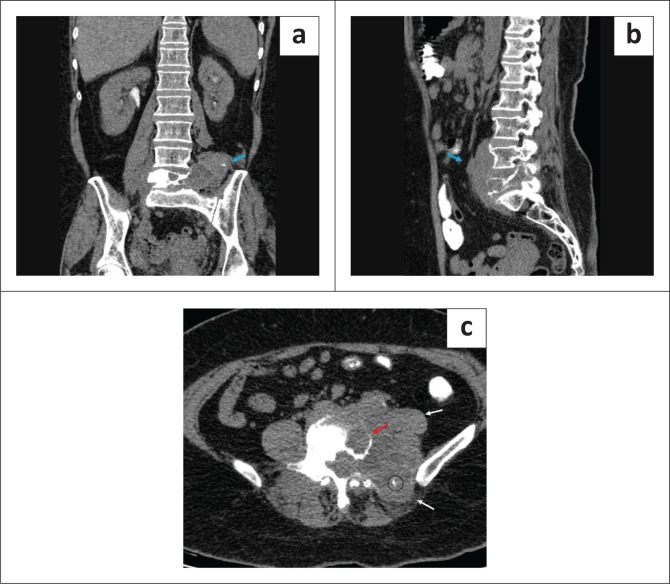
Non-contrast CT scan. An ill-defined soft tissue density lesion is seen along the anterior aspect of the inferior endplate of the L3 vertebra to the anterior aspect of the S1 vertebral body and extending laterally from the L5 vertebral body to the right and left paravertebral regions, predominantly on the left side (blue arrow) (a–b). The L5 vertebral body appears sclerotic and shows an expansile destructive lytic lesion involving the vertebral body with complete destruction of the left lateral vertebral body, left transverse process, left pedicle, left lamina of vertebral arch and left superior articular process (red arrow). Posteriorly, the lesion is seen extending between the left iliac crest and left transverse process of the L4 vertebra and is seen compressing the left posterior paraspinal musculature. Anteriorly, the lesion is seen displacing the left psoas muscle laterally (white arrows). A few foci of calcification are seen within the soft tissue mass (circle) (c).
